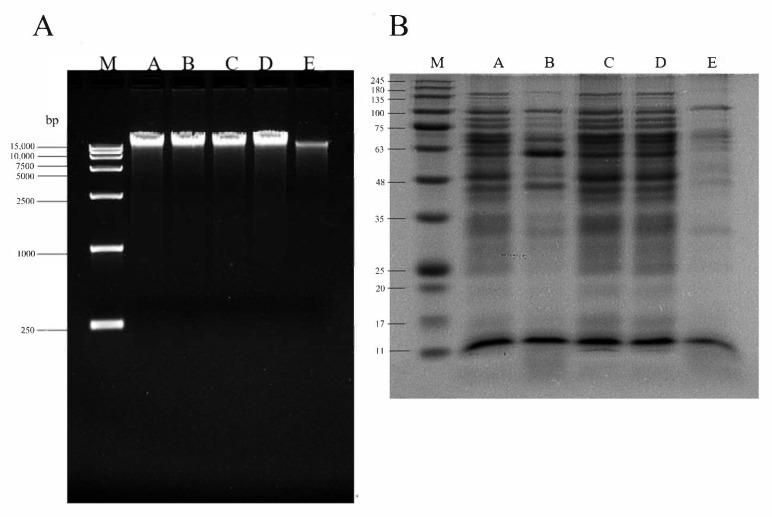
Curcumin-mediated sono-photodynamic treatment induced genomic DNA cleavage and general degradation of bacterial proteins in *Listeria monocytogenes*. (A) Agarose gel electrophoresis analysis of the cleavage of *Listeria monocytogenes* genomic DNA samples. (B) SDS-PAGE profile of *Listeria monocytogenes* total proteins. M: Marker; lane A: Control group without treatment; B: Curcumin-treated group; C: Light-illuminated group; D: Ultrasound-treated group; E: Curcumin-mediated sono-photodynamic treated group.
